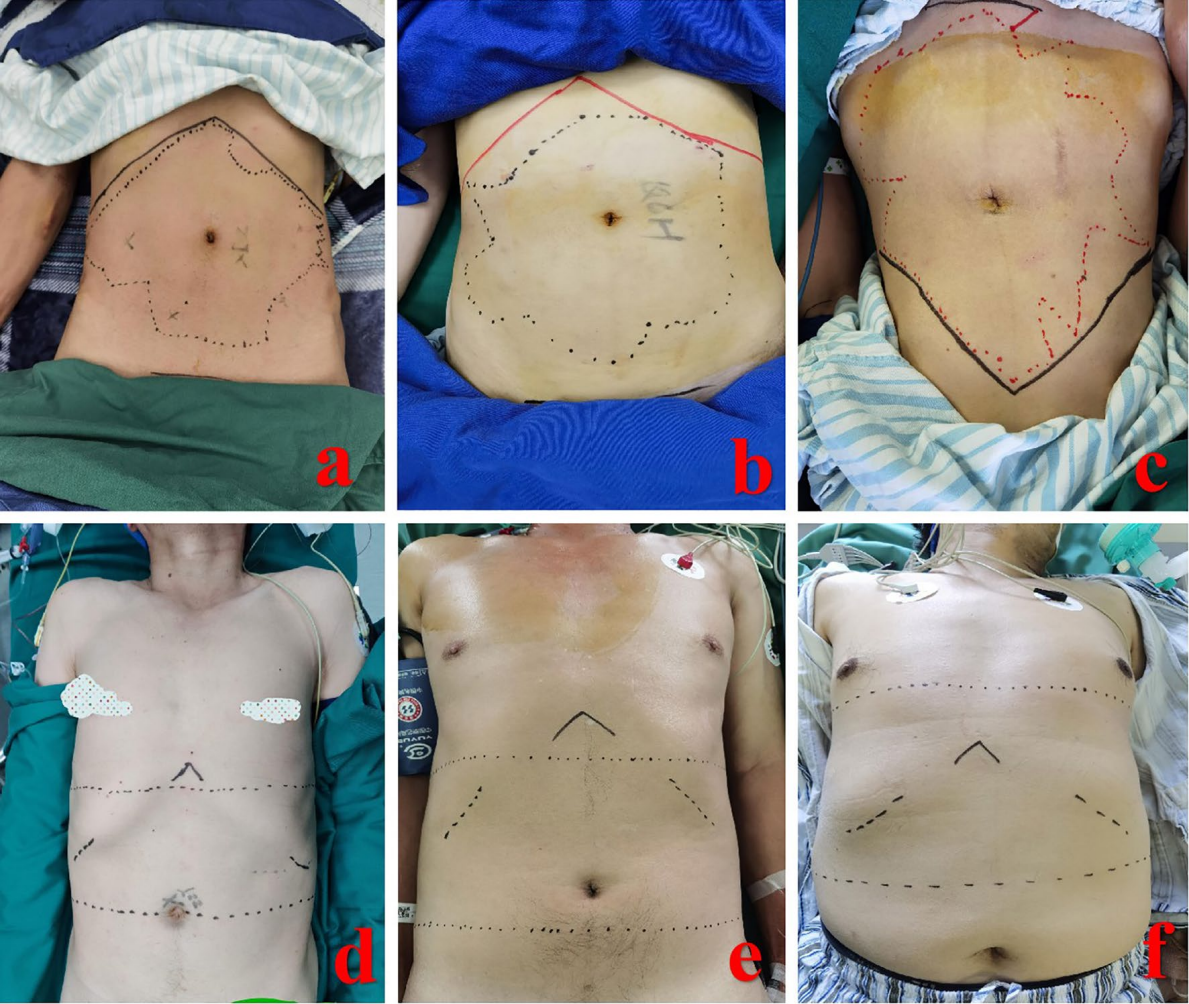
Sensory level of abdominal nerve block and epidural anesthesia with pinprick. (a–c) Sensory level of abdominal nerve block under ultrasound guidance; (d) epidural anesthesia at T11–T12; (e) epidural anesthesia at T12–L1; (f) epidural anesthesia at T9–T10.
